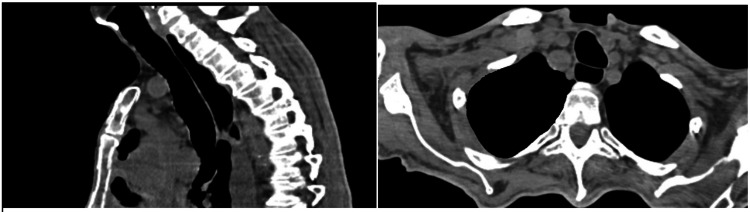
Patient's thoracic computed tomography scans taken one year postoperatively Thoracic computed tomography obtained one year after tracheal mass excision showing a patent tracheal lumen.
